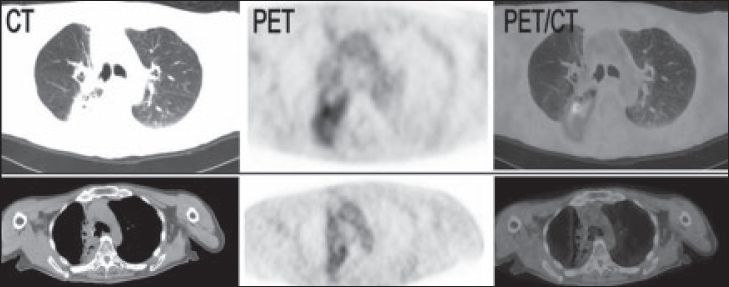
Top images: PET/CT abnormalities secondary to radiation pneumonitis. Bottom images: Resolving radiation changes 5 months later (Reprinted with permission of reference).[39]
